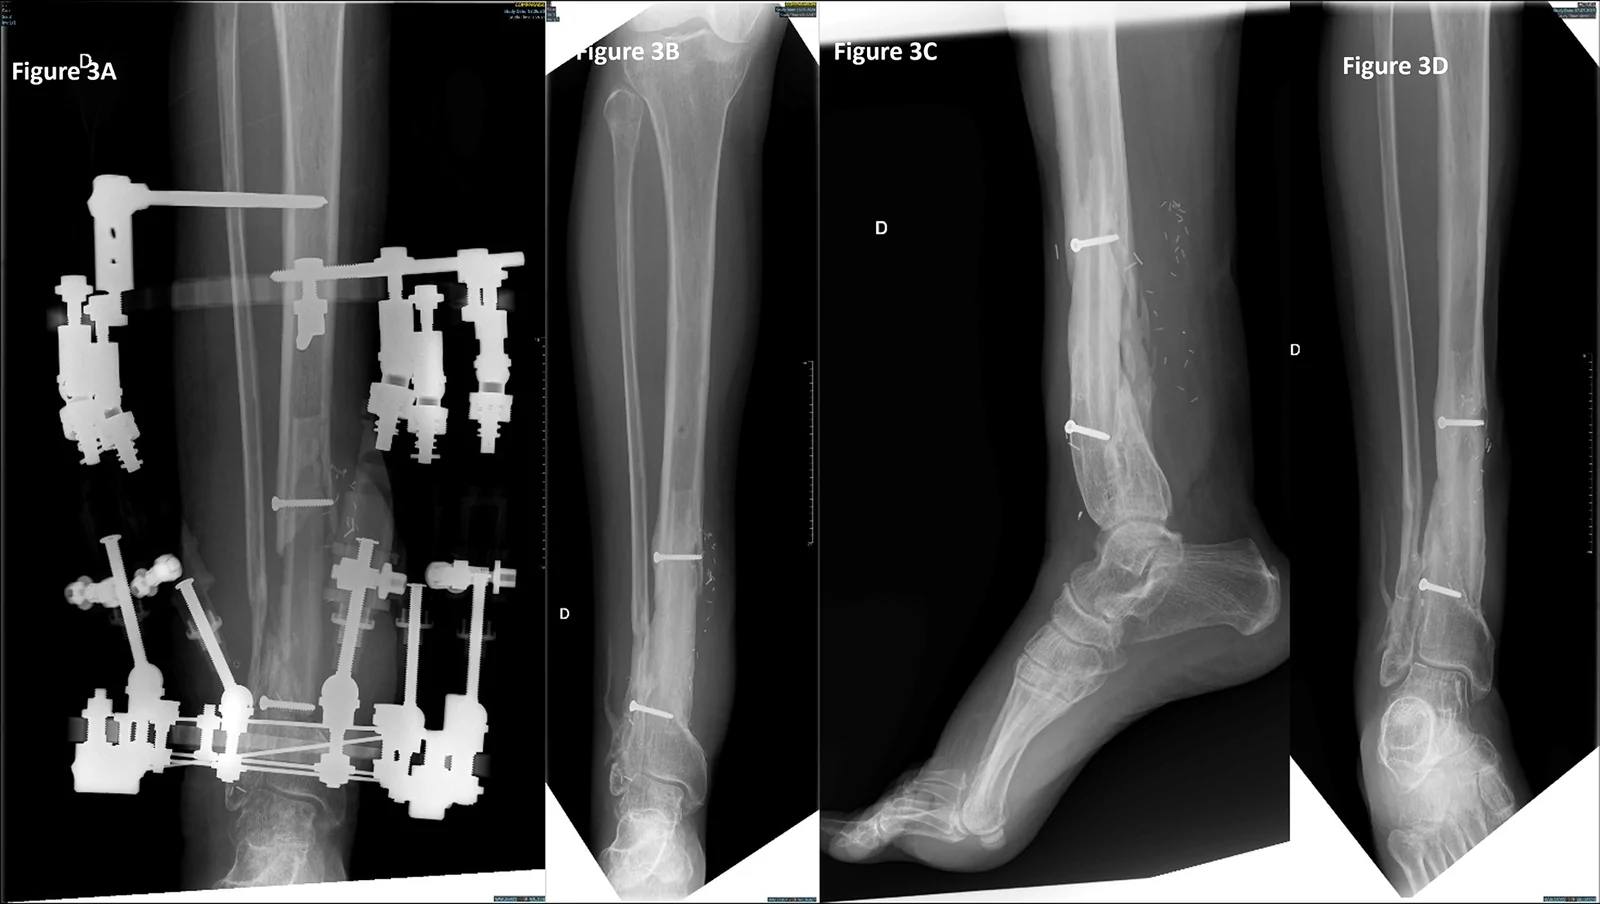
Serial radiographic evolution following vascularized fibular grafting (VFG) for infected non-union. (A) Immediate postoperative radiograph after VFG reconstruction. (B) Radiograph obtained 13 months after surgery showing progressive graft incorporation. (C, D) Radiographs obtained 21 months after VFG demonstrating advanced graft hypertrophy, cortical integration, and bone consolidation.
